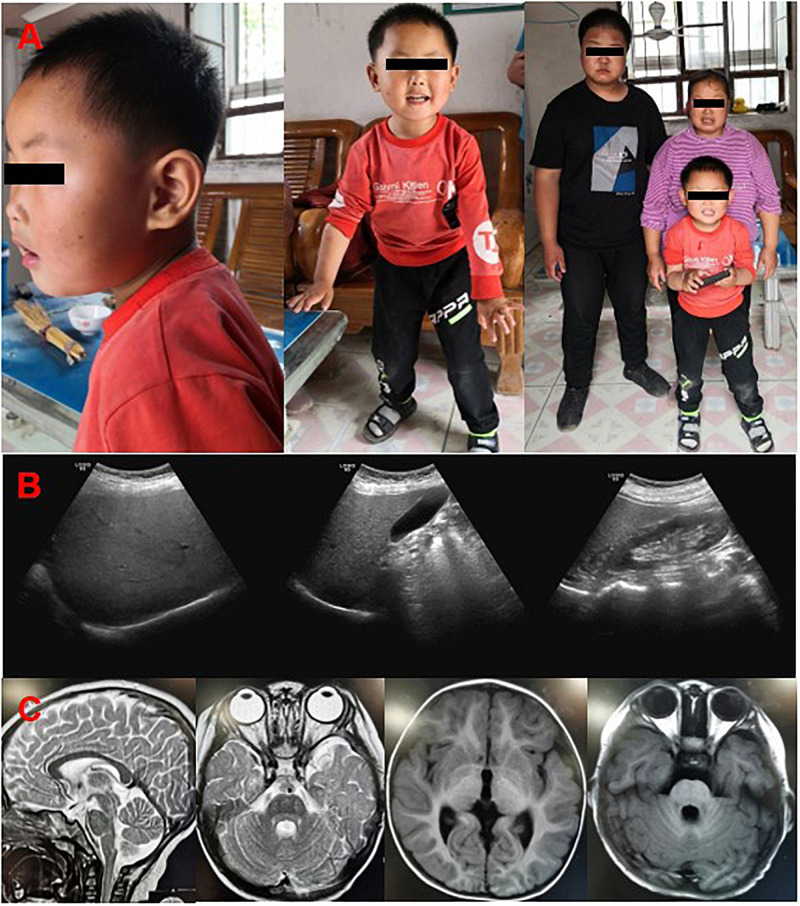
Clinical features of the patients. (A) The pictures of the probands, mother and her two sons in the family by following-up. (B) Color Doppler ultrasound of mother showed that liver section is normal in size, smooth in capsule, dense in liver parenchyma, uniform in distribution, and clear in tube structure. (C) Brain MRI of the younger son showed that no abnormal density lesions were found in the cerebral parenchyma, and cerebrospinal fluid cavity, the ventricle, sulcus, and cleft lobes were normal, the brain gyrus was clear, and the midline structure was centered.
